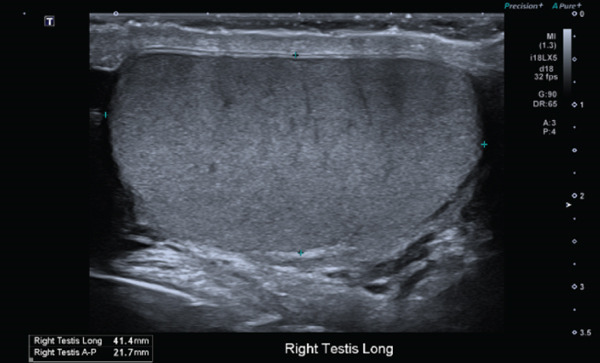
The right testicle on ultrasonography measures 41 × 22 × 29 mm, 14 cc and appears sonographically normal.
